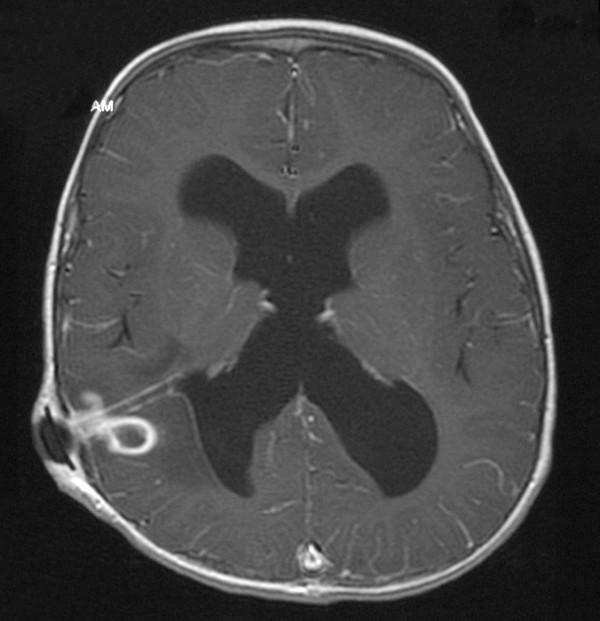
MRI (T1 + contrast): showing a small ring-enhancing lesion with mild surrounding edema adjacent to the ventricular catheter and ventricular dilatation.
